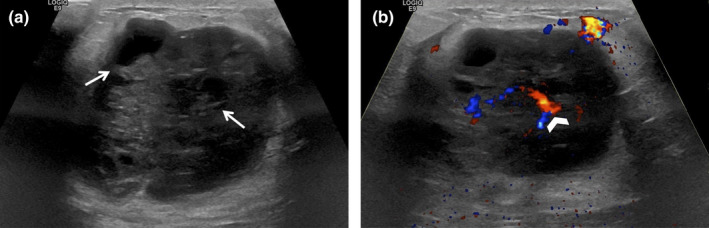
37‐year‐old woman presented to the emergency room with a breast lump, clinically concerning for an abscess. Ultrasound was performed with an ML6‐15 MHz transducer with the frequency set to 9 MHz, and the colour scale was set to 5 cm/s. Targeted ultrasound showed a 4.8 cm heterogeneous hypoechoic mass with internal cystic spaces (arrows) (a). Colour image showed internal vascularity (chevron) but a drainable fluid component was not identified (b). A recommendation was made for further completed diagnostic workup for malignancy in the breast clinic and breast imaging. The evaluation was completed in the breast imaging department 18 days later, where a biopsy was performed confirming invasive ductal carcinoma, with a Nottingham grade of 3/3.
